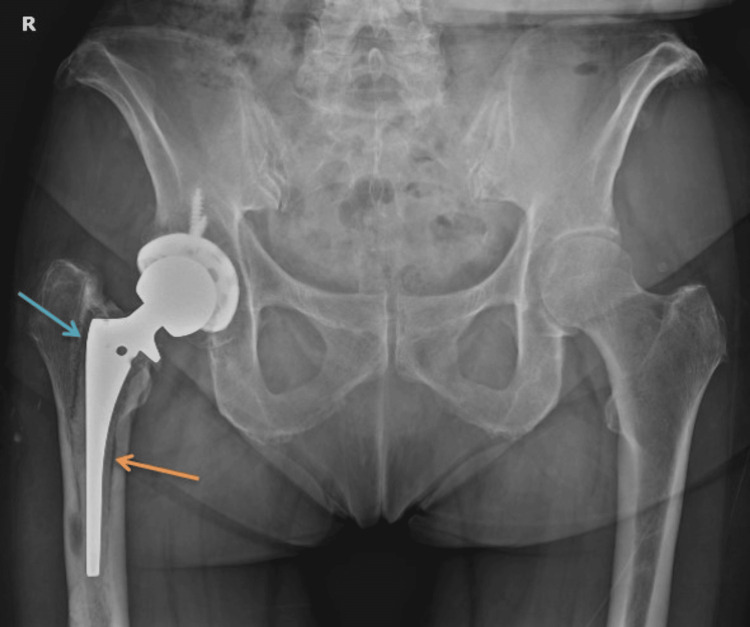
Anteroposterior X-ray of the pelvis. Arrows indicate implant-cement interface. Blue arrow demonstrates radiolucency between the stem and cement mantle in Gruen zone 1. Orange arrow demonstrates radiolucency between cement mantle and bone.
